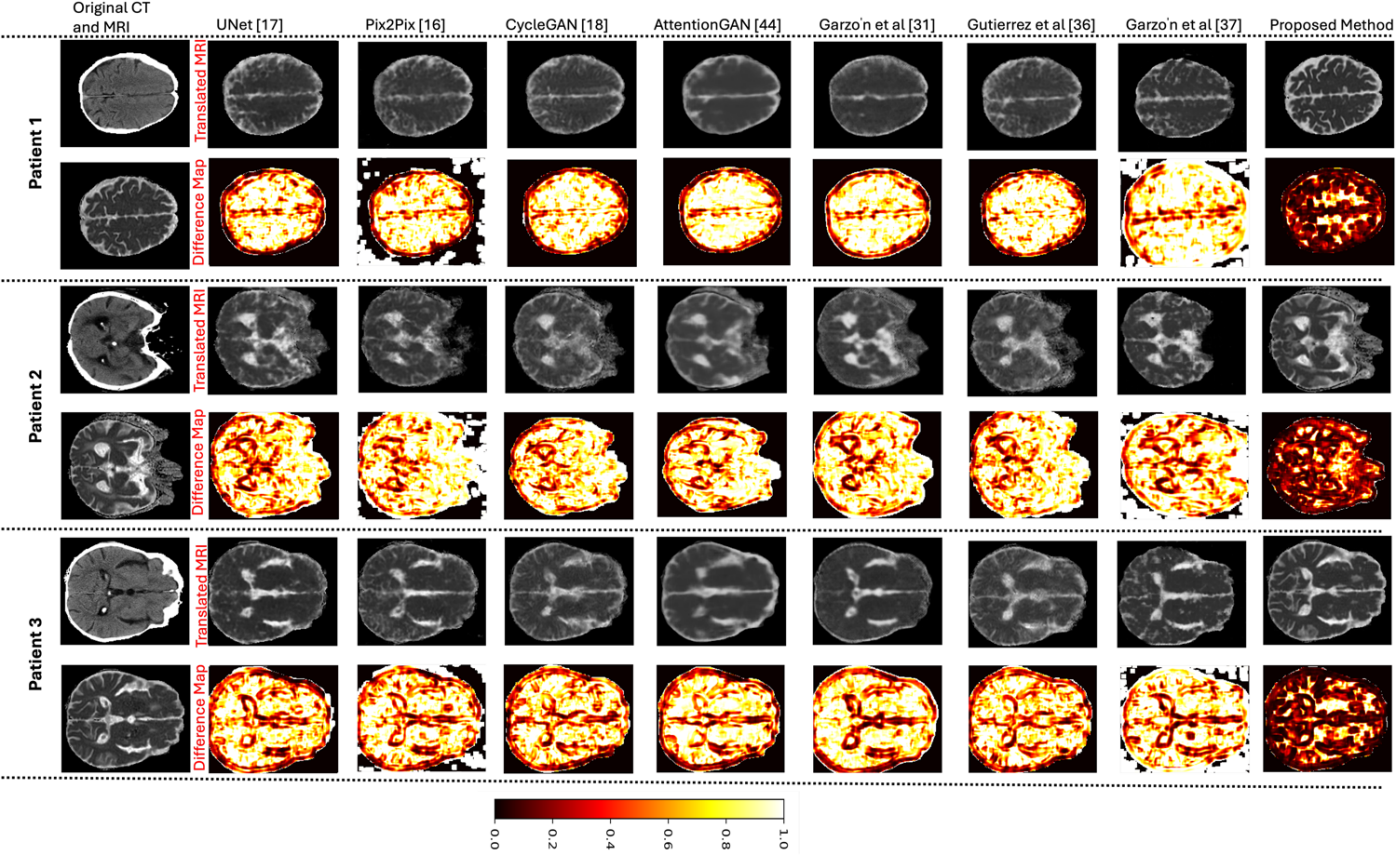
The qualitative evaluation results of the UNet [17], Pix2Pix [16], CycleGAN [18], AttentionGAN [43], Garzón et al. [31], Gutierrez et al. [36], Garzón et al. [37] and Proposed Method for the translation of CT images into ADC MRI images in three normal cases. Difference maps for each methods are displayed using a black-red-yellow colormap, where black = no difference (0) and yellow = complete difference (1)
